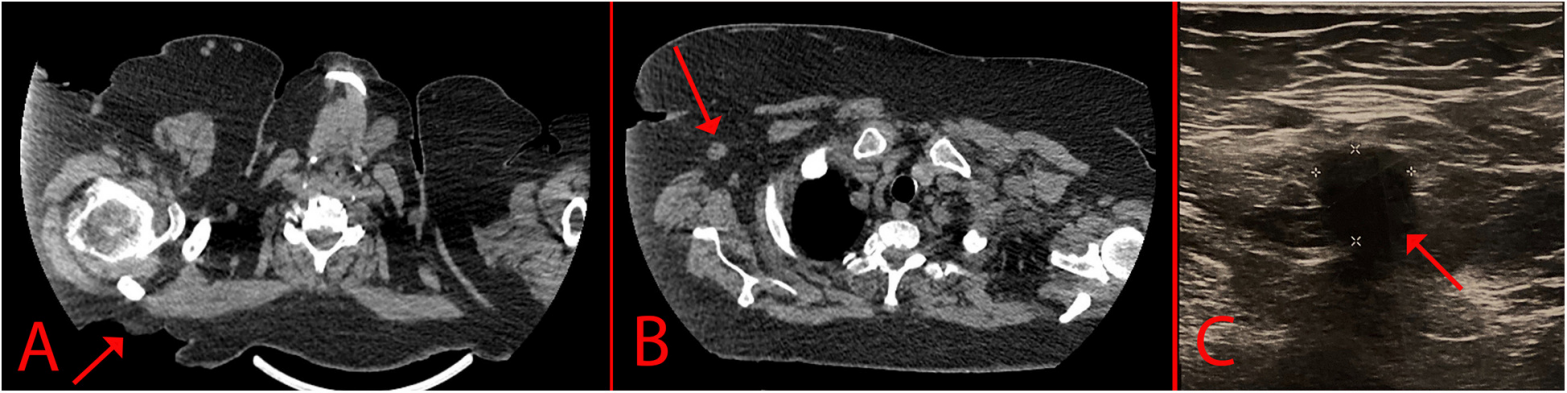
Imaging of the lesion. (A) – CT scan that indicates a skin defect; (B) – Enlarged axillary lymph node; (C) – USG of the axillary lymph node.
